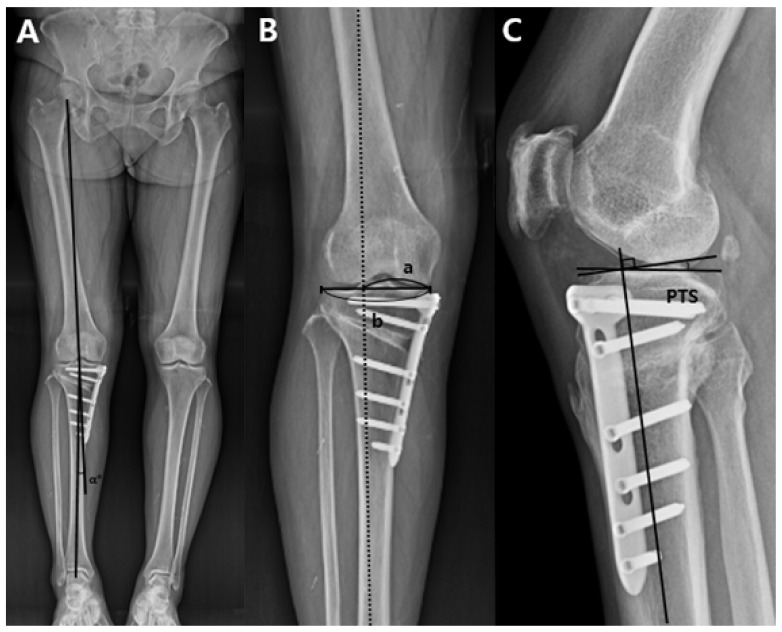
Measurement of the hip-knee-ankle angle, weight-bearing line ratio, and posterior tibial slope on the plain radiographs. (A) The hip-knee-ankle angle (α°) is formed by the first line drawn from the center of the femoral center to center of the tibial spine and the second line drawn from the center of the tibial spine of knee joint to the center of the superior articular surface of the talus in the ankle joint. The angle made by the intersection of these two lines was defined as the hip-knee-ankle angle. (B) The weight-bearing line was drawn from the center of the femoral head to the middle point of the superior articular surface of the talus on the full-length lower extremity standing radiograph. A tibial plateau line from the medial edge to the lateral edge of the proximal tibia on the joint surface was drawn thereafter. Weight-bearing line ratio (a/b) was calculated as the ratio of the distance from the medial edge of the proximal tibial plateau to the intersection of the weight-bearing line and the proximal tibial plateau line (a) to the entire length of the proximal tibial plateau line (b). The medial tibial edge was 0%, and the lateral tibial edge was 100%. (C) The posterior tibial slope is formed by the perpendicular line to the tibial shaft axis and the medial tibial plateau line on the true lateral view. posterior tibial slope = posterior tibial slope.
